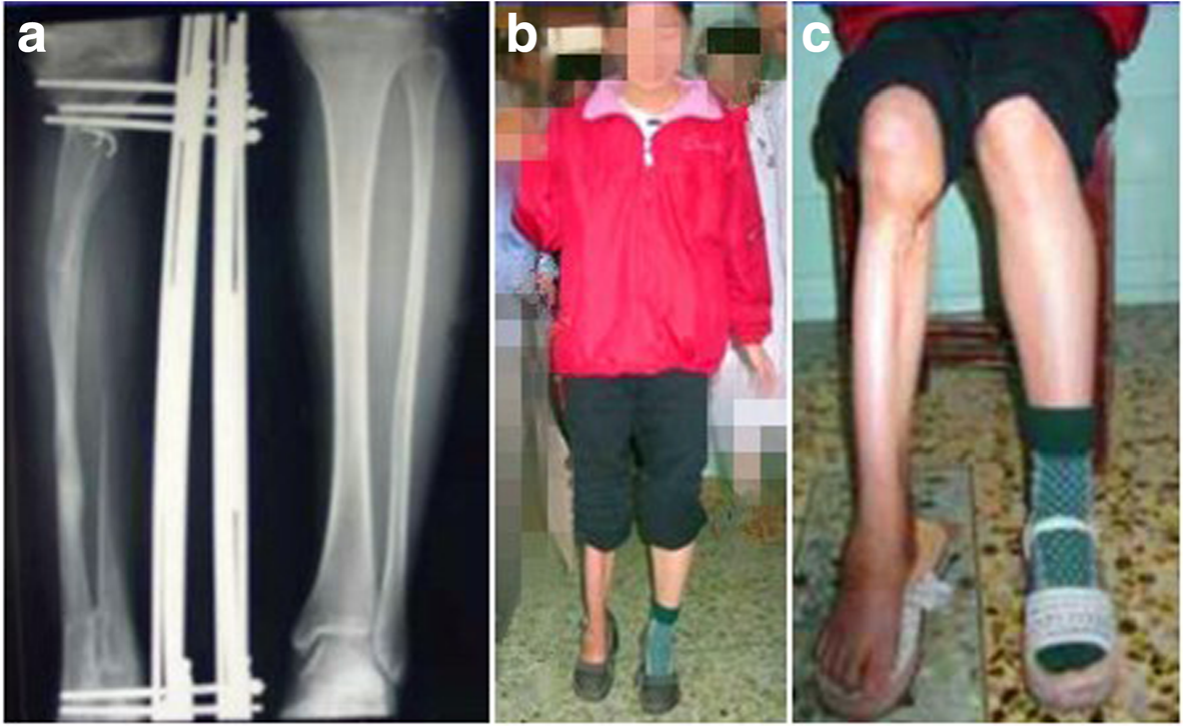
The X-ray film reveals a total lengthening of 18.0 cm was achieved (a), and she can walk well without walking aids or braces (b), and the knee and ankle’ function were near normal at the last follow-up (c)
